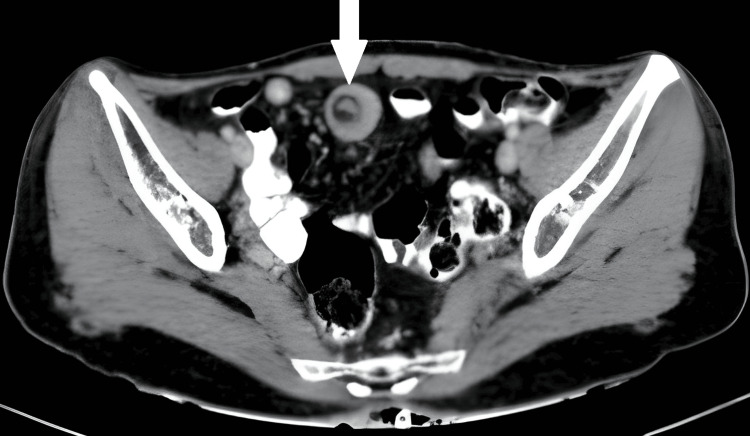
CT scan of the abdomen and pelvis The CT scan of the abdomen and pelvis showed a submucosal lipoma, coming about in small bowel intussusception and partial bowel obstruction, as indicated by the white arrow. CT: computed tomography.
